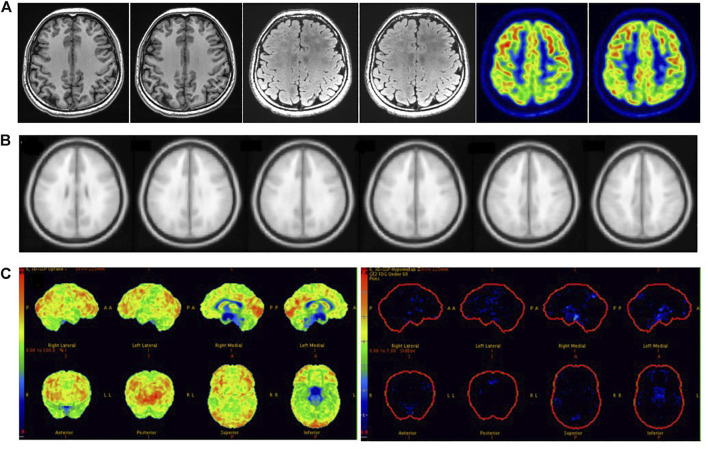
Female, 21 years old, right frontal lobe epilepsy. (A) No structural lesions causing seizures were found at MRI 3.0 T epilepsy-protocol. ^18^F-FDG PET obtained abnormal decreased metabolism in the left frontal, parietal and temporal lobes. (B) SPM obtained abnormal metabolism in left temporal lobe while *p* < 0.005 and K > 100. (C) 3D-SSP obtained abnormal metabolism in right frontal, double parietal and double occipital lobes. Further SEEG examination obtained the epilepsy foci originated from right frontal lobe. There had been no seizures for 3 years after surgical resection.
